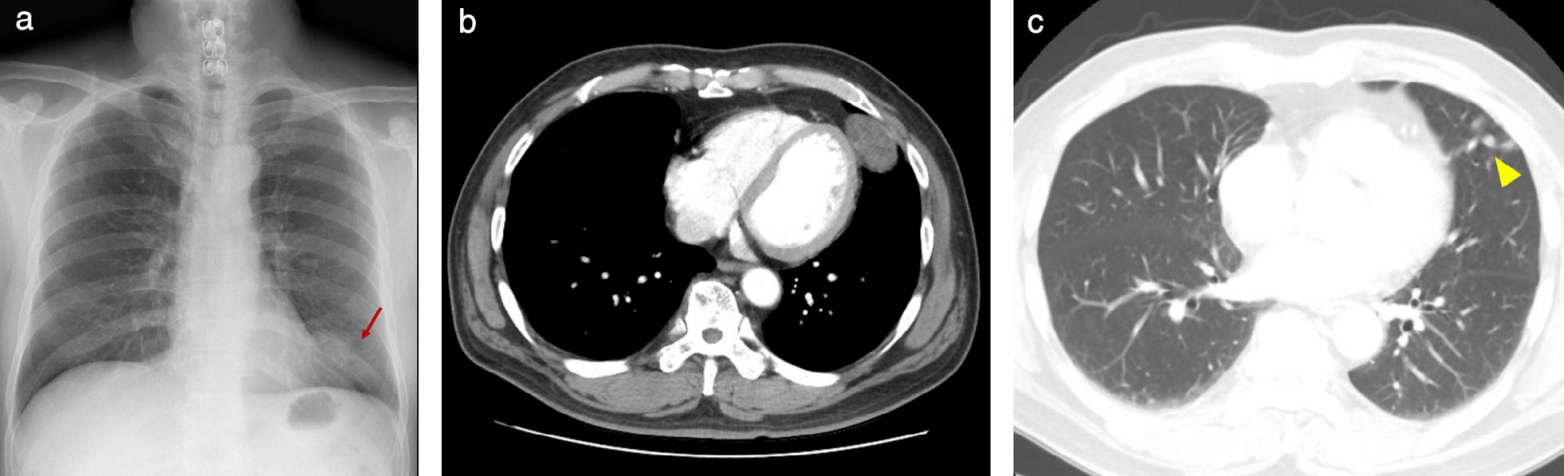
Chest images. (a) Chest X‐ray revealed a mass‐like opacity in the lower zone of the left lung (arrow). (b) Computed tomography (CT) scan of the chest showed an inhomogenous well‐defined mass with internal low attenuations in the left lower paracardiac area. (c) CT scan of the chest showed multiple subpleural nodules in the upper lobe of the left lung (arrowhead).
